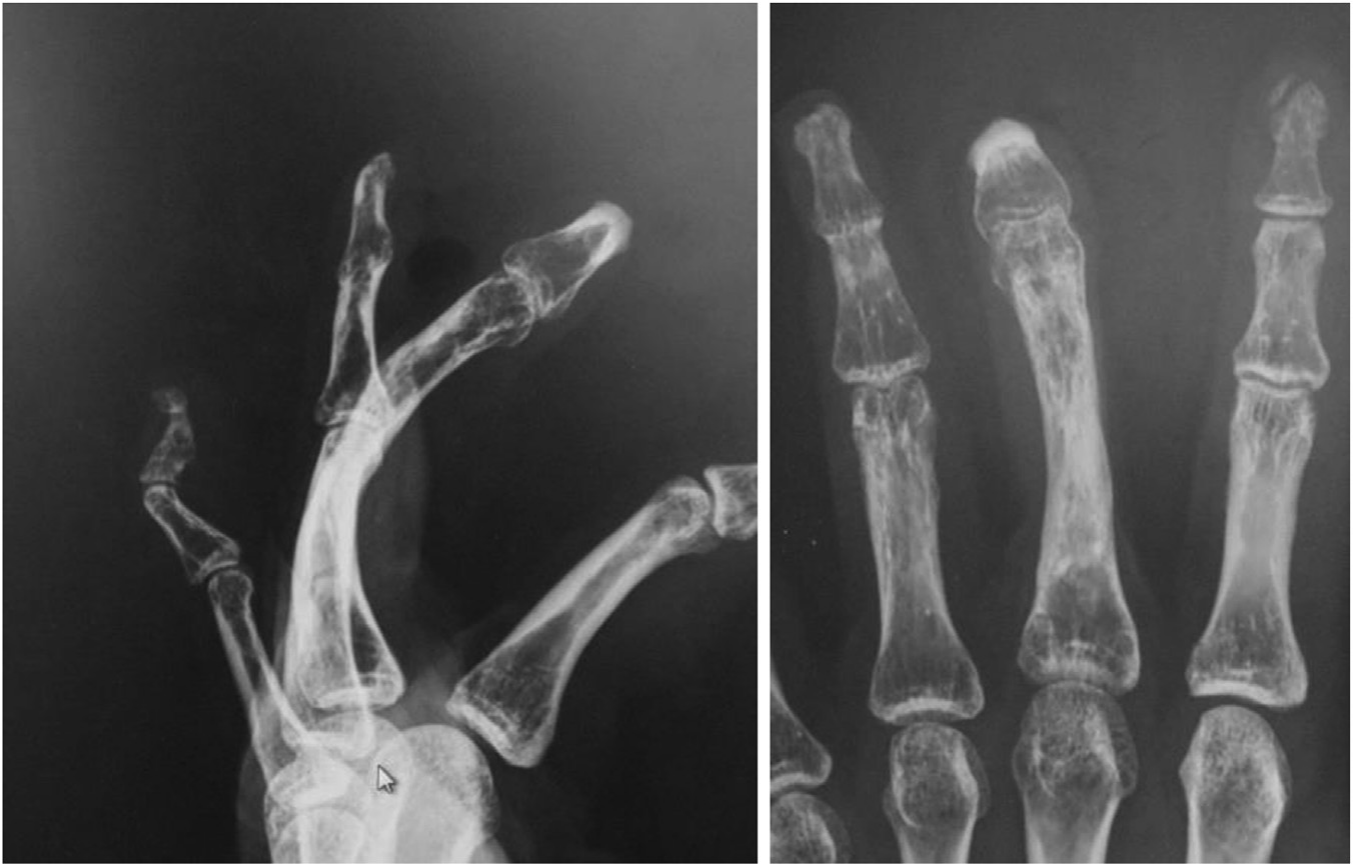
(Left) Lateral view of the X-ray of Case 1, 5 years after the wire removal. (Right) Anterior posterior view of the X-ray.
